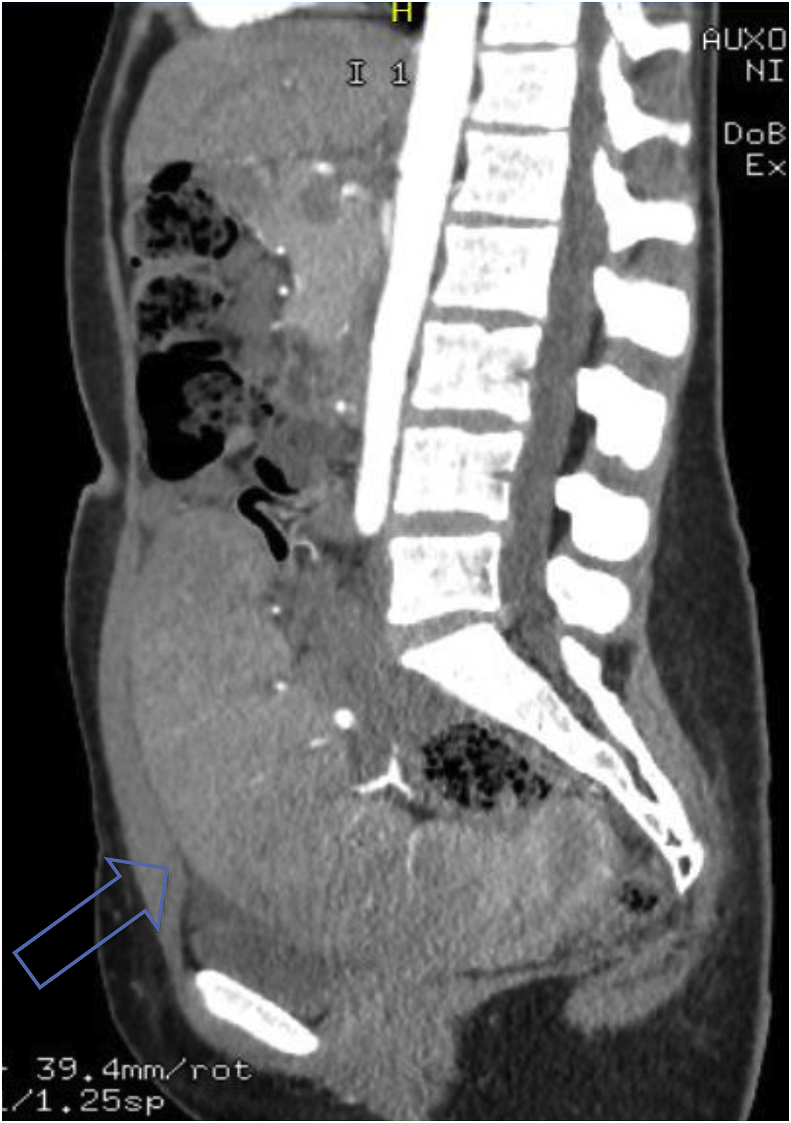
Contrast-enhanced sagittal CT image showing cranio-caudal extension of the spleen.
